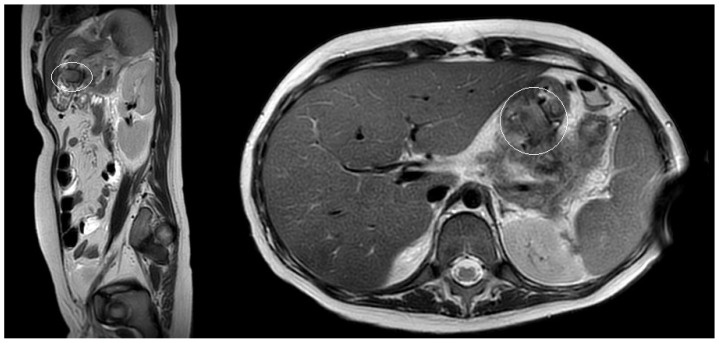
Patient n°3 MRI. Reduction of the walled-off necrosis; the device is in place (in the white circle).
